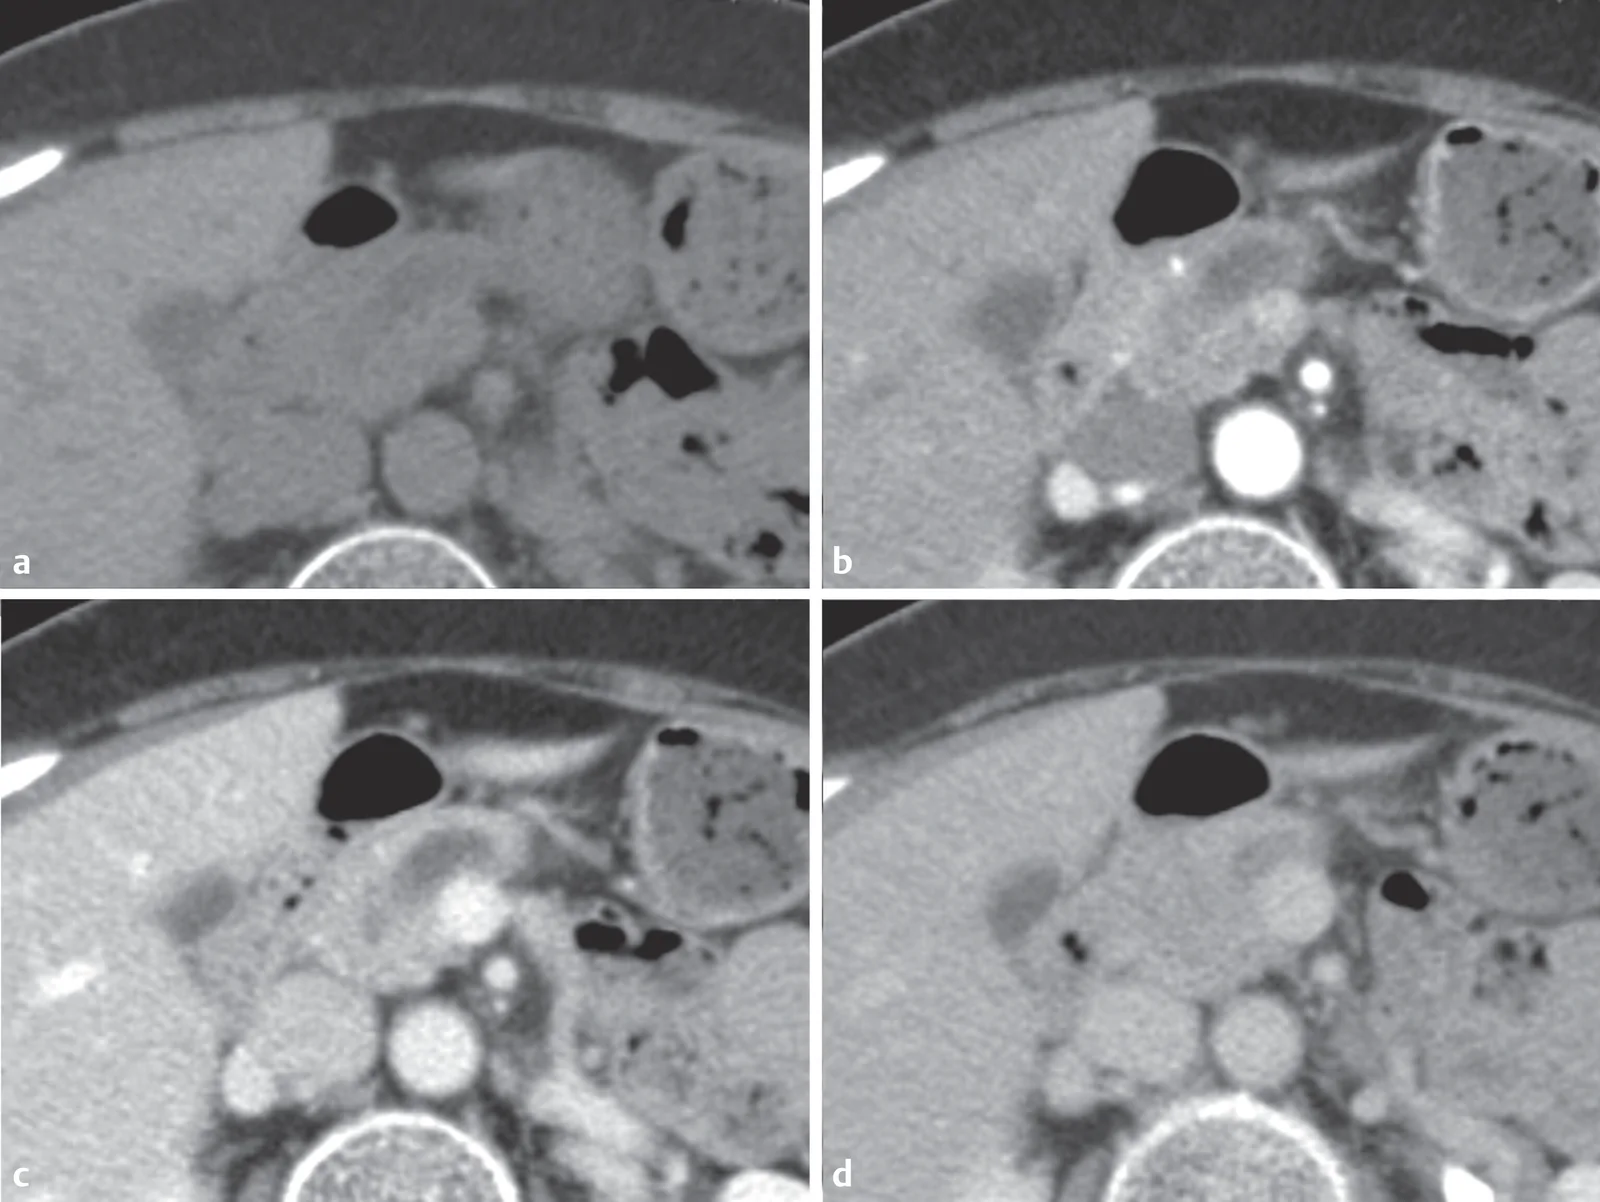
Dynamic CT showing the dilation of the MPD without an
